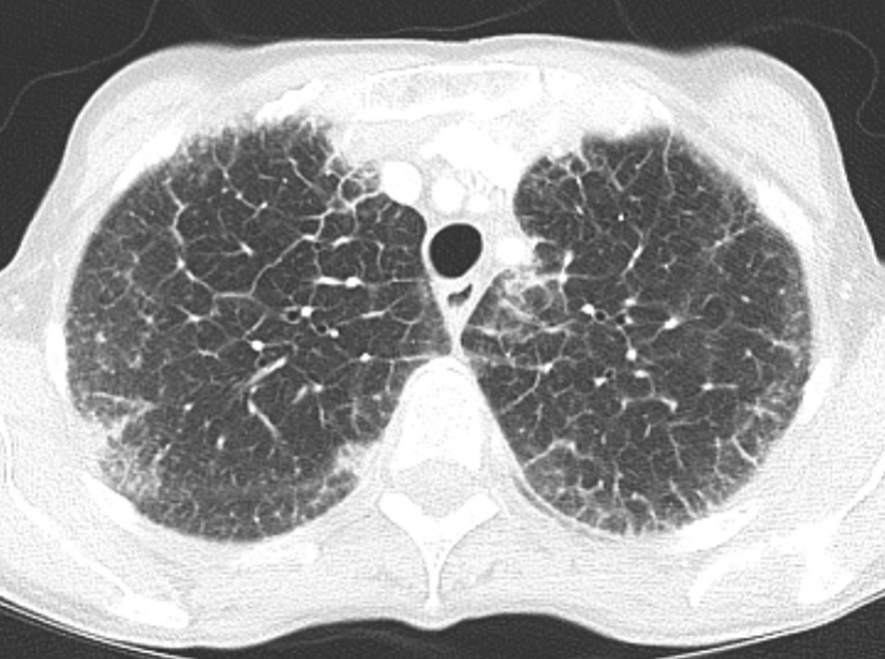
CT scan of the chest. Lung windows demonstrate thickened interstitial septal lines.
